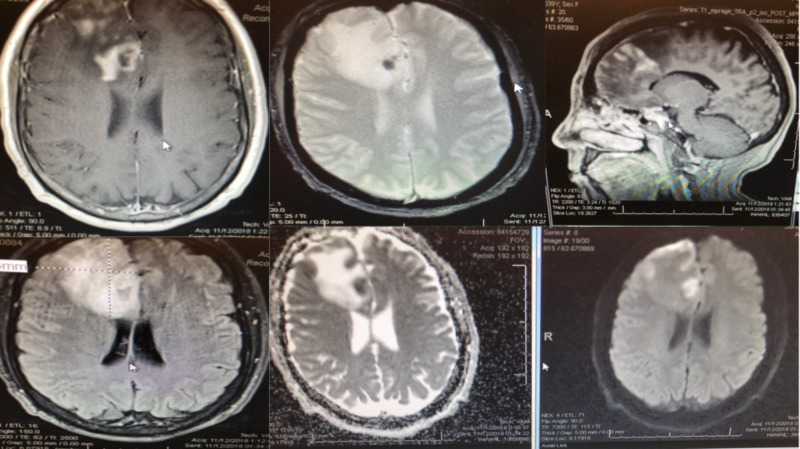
Preoperative MRI brain showing small areas of hemorrhage and diffusion restriction in medial frontal lobe. Top Left: Axial T2, Bottom Left: Axial fluid attenuated inversion recovery (FLAIR), Top Middle: Axial gradient echo (GRE), Bottom Middle: Axial apparent diffusion coefficient (ADC), Top Right: Sagittal T1 with contrast, Bottom Right: Axial diffusion weighted imaging (DWI)
